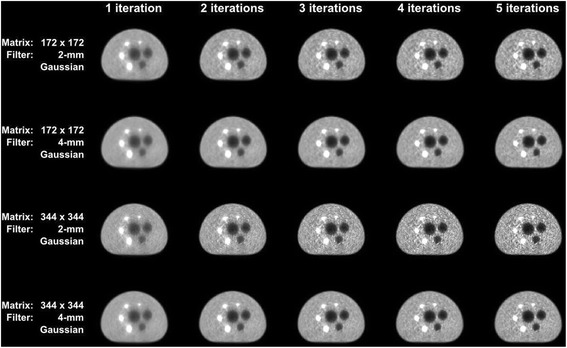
Images of the first five iterations reconstructed with 3D OP-OSEM for different matrix and Gaussian filter sizes and using CT-based AC. Note: increasing the number of iterations is associated with increasing lesion sharpness while also increasing background noise. This also applies when increasing the image matrix size from 172 x 172 to 344 x 344
